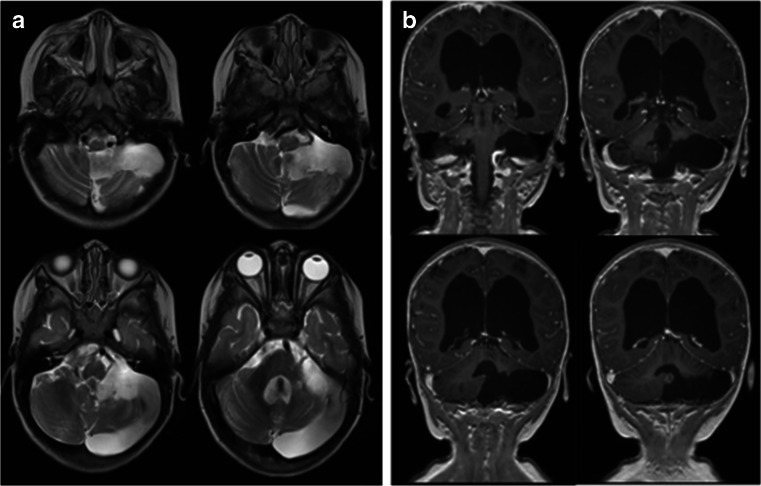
Case 2. Three years post-operative MRI (a T2-weighted axial view, b, T1-weight coronal image after contrast) showing no signs of residual or recurrent tumorCase 3. This is a 4-year-old girl who presented with intermittent headaches with fever since a year ago. Due to increasing episodes of “chemical meningitis,” she had head CT and MR, she had CT and subsequent MRI which showed a large non-enhancing mass in the midline ventral to the brain stem at the pontomedullary junction (Fig. 9). Pre-operative neurological examination was normal, however. Pre-operative laryngoscopy showed normal vocal cord movements. She had a PF craniotomy with the bone flap primarily on the left occipital bone crossing the midline and crossing the foramen magnum (Fig. 10a). The CMF and CPA were entered by lifting the cerebellar tonsil and hemisphere of the left side (Fig. 10b). Medially to the 9th and 10th nerves and also to the 7th and 8th nerve complex was the cloudy capsule of an epidermoid (Fig. 10c). Clearly, debris from the epidermoid contents was noted floating in the CSF space. The main approach was through the space below the 10th nerve and another above the 9th nerve. The lesion was removed from the space between the basilar artery and the ventral surface of the brain stem (Fig. 10d). Postoperatively, she developed a left vocal cord palsy, needing G-tube. She started to take oral feeding by 3 months and G-tube was removed 6 months postoperatively. At the last examination 4 years following the surgery, she had normal neurological function and follow-up laryngoscopy showed normal vocal cord function. No signs of epidermoid recurrence were noted on follow-up MR (Fig. 11)
